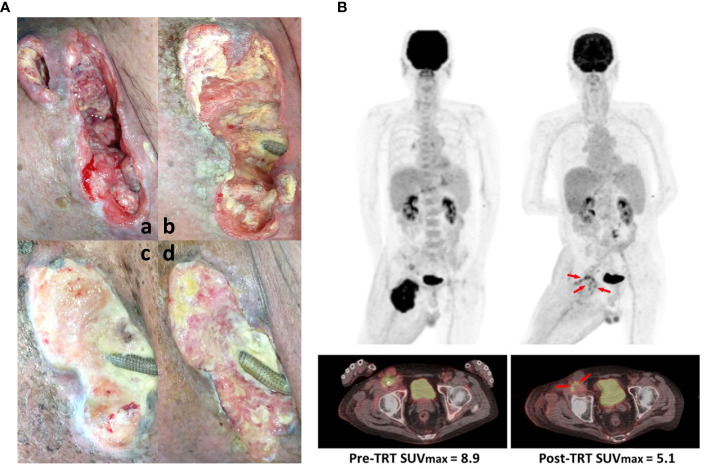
Therapeutic effect of intratumoral immunotherapy combined with local radiation. (A) Primary lesion conditions assessed (a) pre-TRT status, (b) after the first TRT course, (c) after the second TRT course, and (d) after the whole TRT period. (B) The tumor mass shrank with remarkable SUVmax reduction of 42% on FDG positron emission tomography (PET)-Scan. Tumor sizes before any treatment (left panel) and after the complete TRT period (right panel) were assessed using PET.
